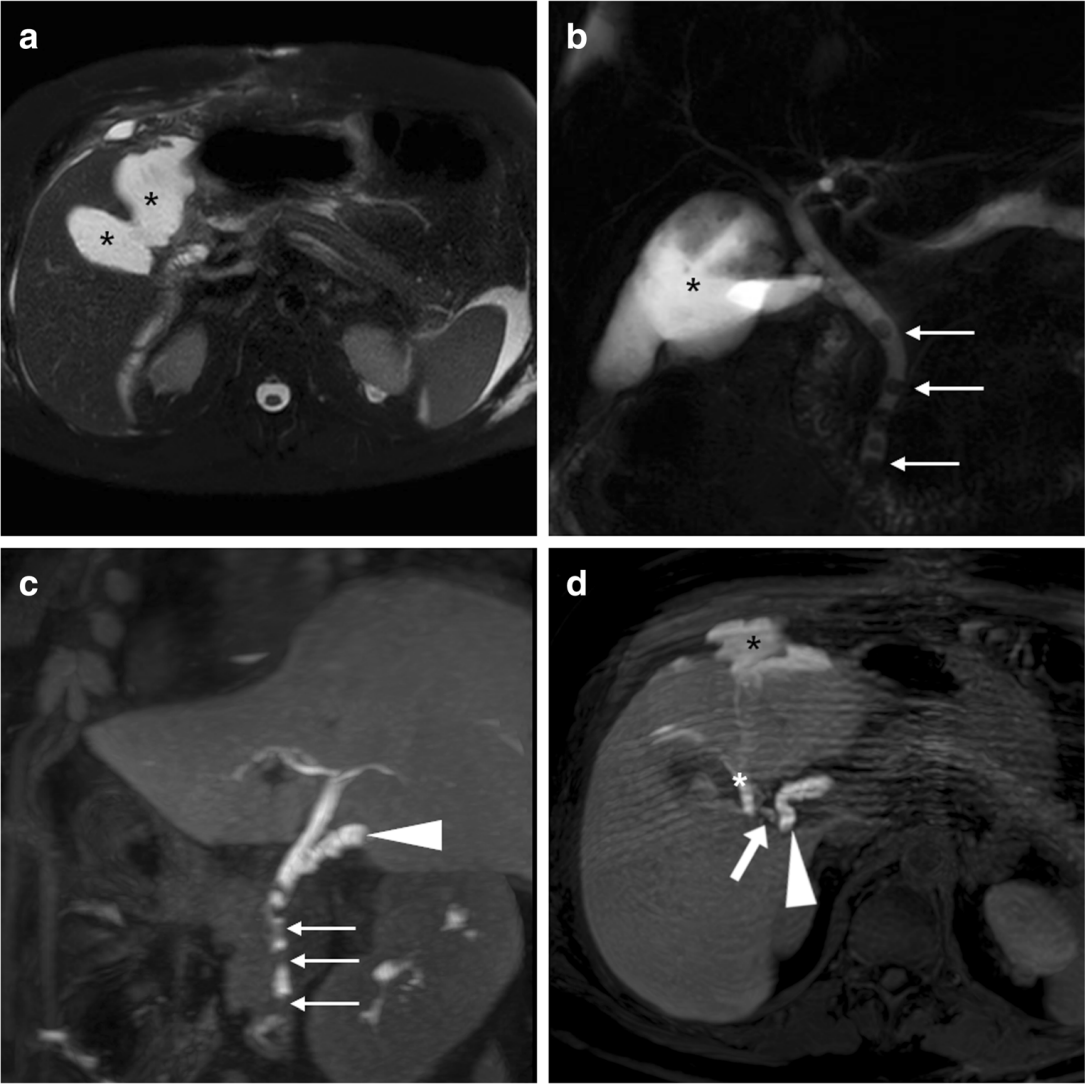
On the 7th postoperative day after laparoscopic cholecystectomy, urgent MR including fat-saturated T2-weighted (a) and MRCP (b) showed sizeable biloma (*) extending ventrally from the gallbladder fossa, some peritoneal fluid and mildly dilated CBD containing multiple millimetric filling defects consistent with stones (*thin arrows* in b). Gadoxetic acid-enhanced MRCP confirmed residual choledocholithiasis (*thin arrows* in c) and allowed detecting a small biliary leak (*arrow* in d) from the cystic duct remnant (*arrowheads*), causing opacification of the biloma (*). Endoscopic treatment included sphincterotomy and placement of a self-expanding metal stent
